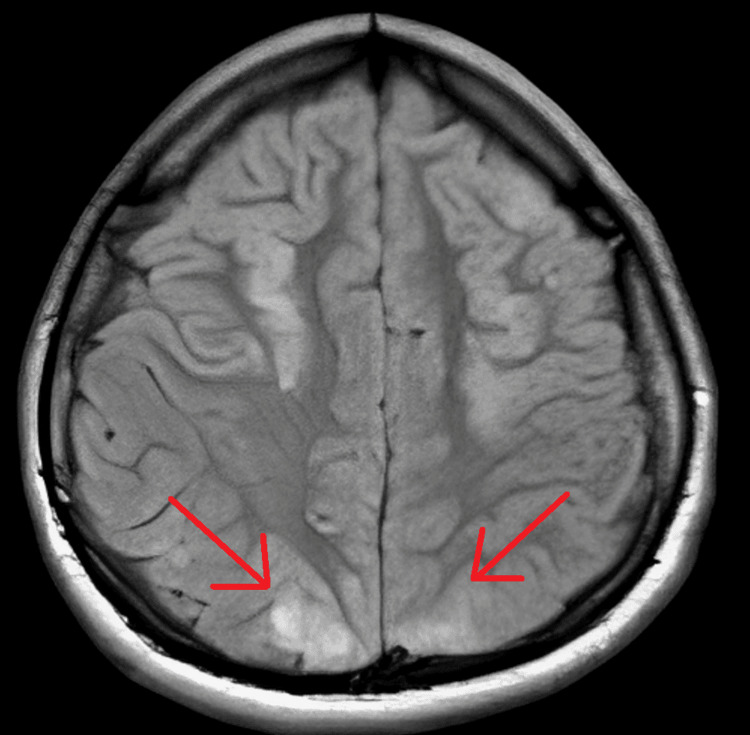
Cranial magnetic resonance imaging (FLAIR sequence) Bilateral posterior symmetric hyperintensities consistent with vasogenic edema (red arrows) FLAIR: fluid-attenuated inversion recovery
